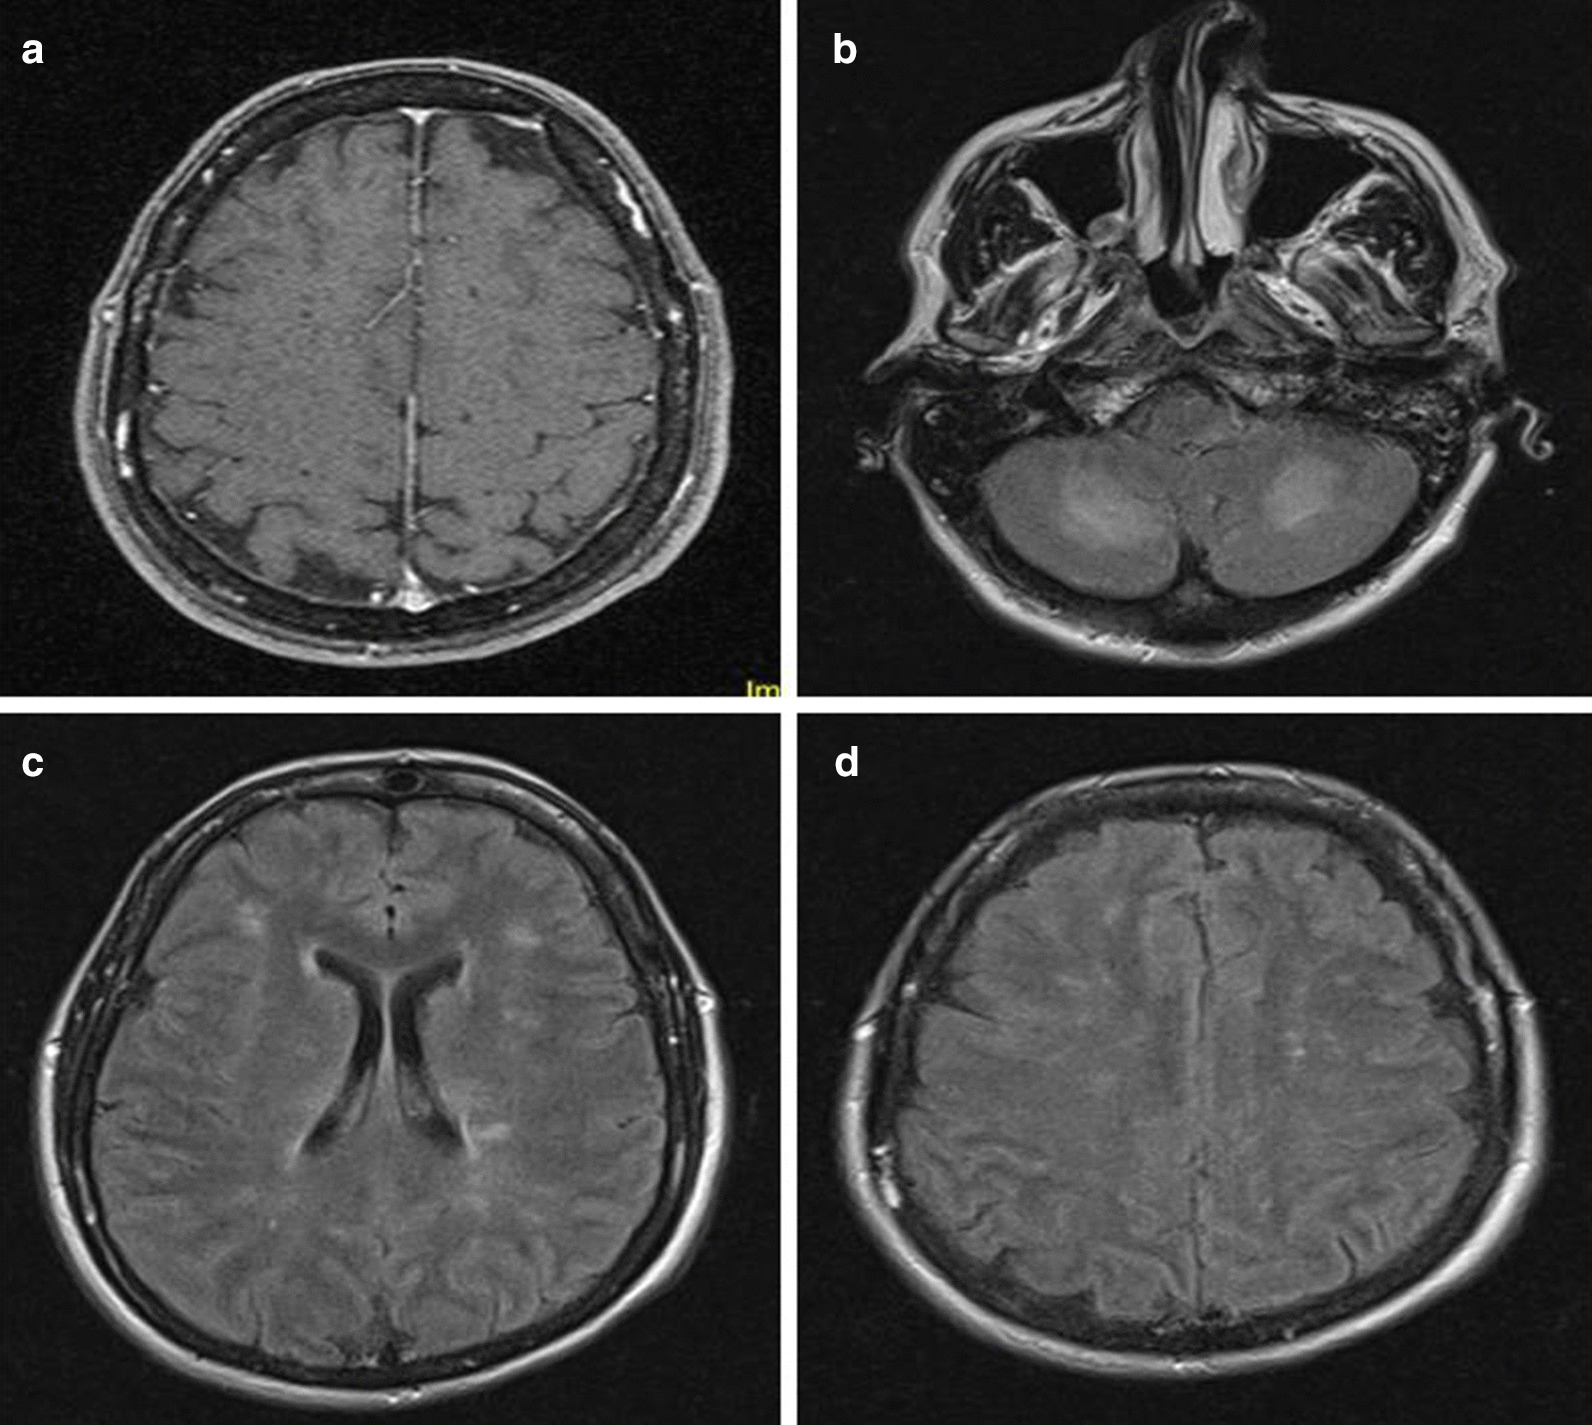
Images from the first MRI scan. a T1-weighted image showing meningeal post-contrast enhancement. b FLAIR image demonstrating bilateral cerebellar hyperintense lesions in both hemispheres. c FLAIR image showing periventricular hyperintensity and multiple white-matter hyperintense lesions. d FLAIR image demonstrating multiple juxtacortical white-matter lesions
